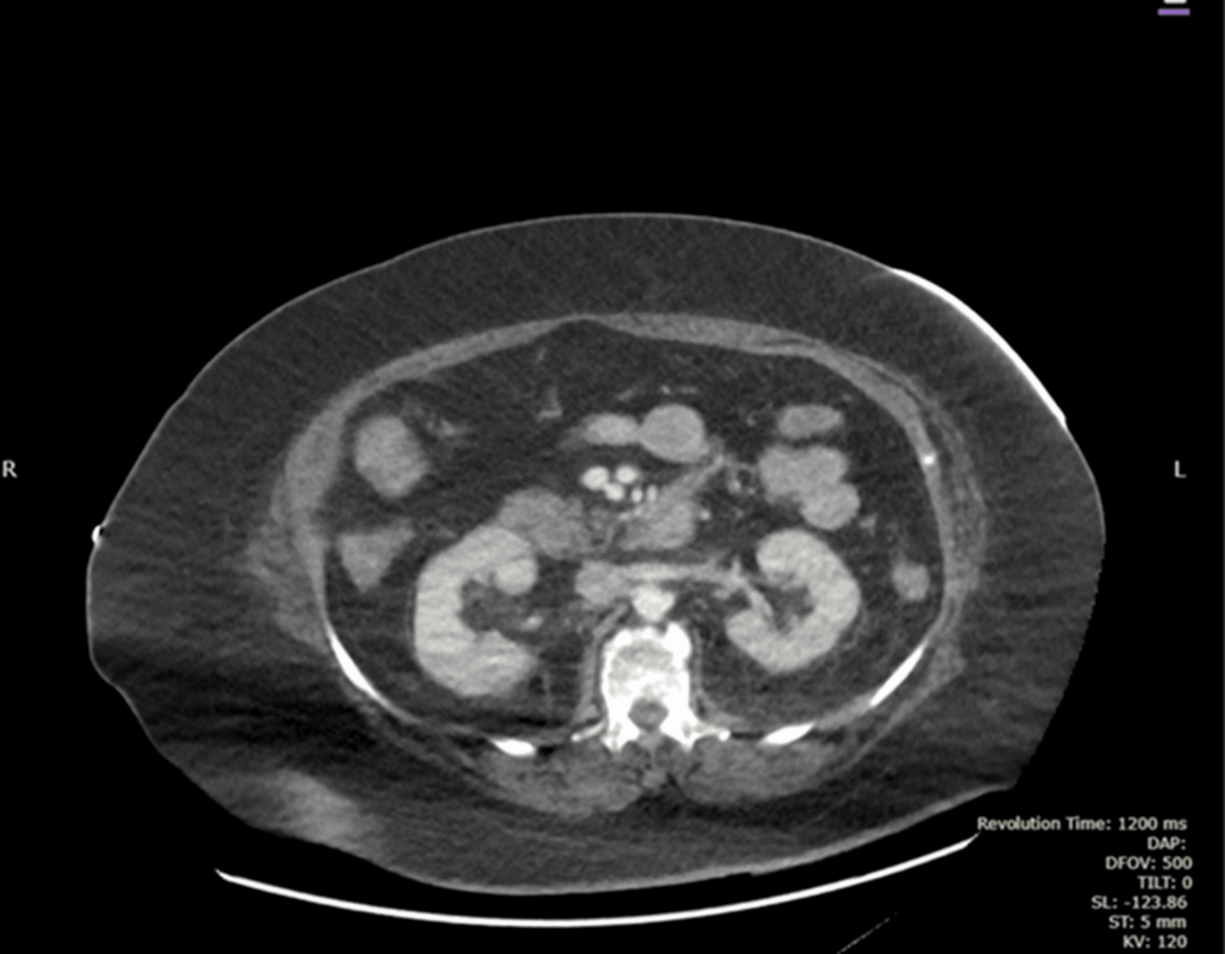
CT Abdomen with Contrast Pancreas is normal in size with normal shape
